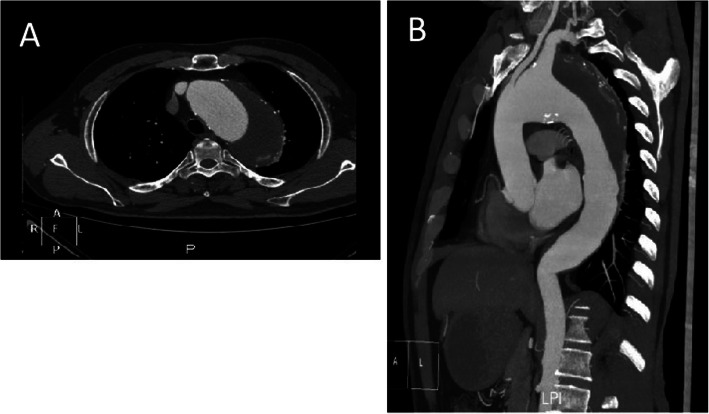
a, b: Preoperative CTA
